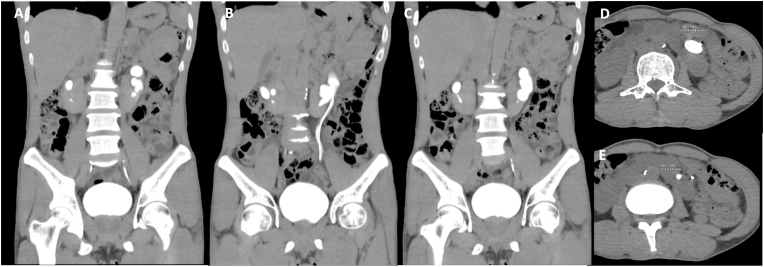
Preoperative imaging findings in a patient with a horseshoe kidney and complex renal stones. (A–C) Coronal contrast-enhanced CT urography images demonstrating a horseshoe kidney with fused lower poles and multiple left renal stones, including the largest stone measuring 21.9 × 18.2 × 13.2 mm. (D–E) Axial images confirming high-density renal stones (1297 HU) within the left collecting system.
